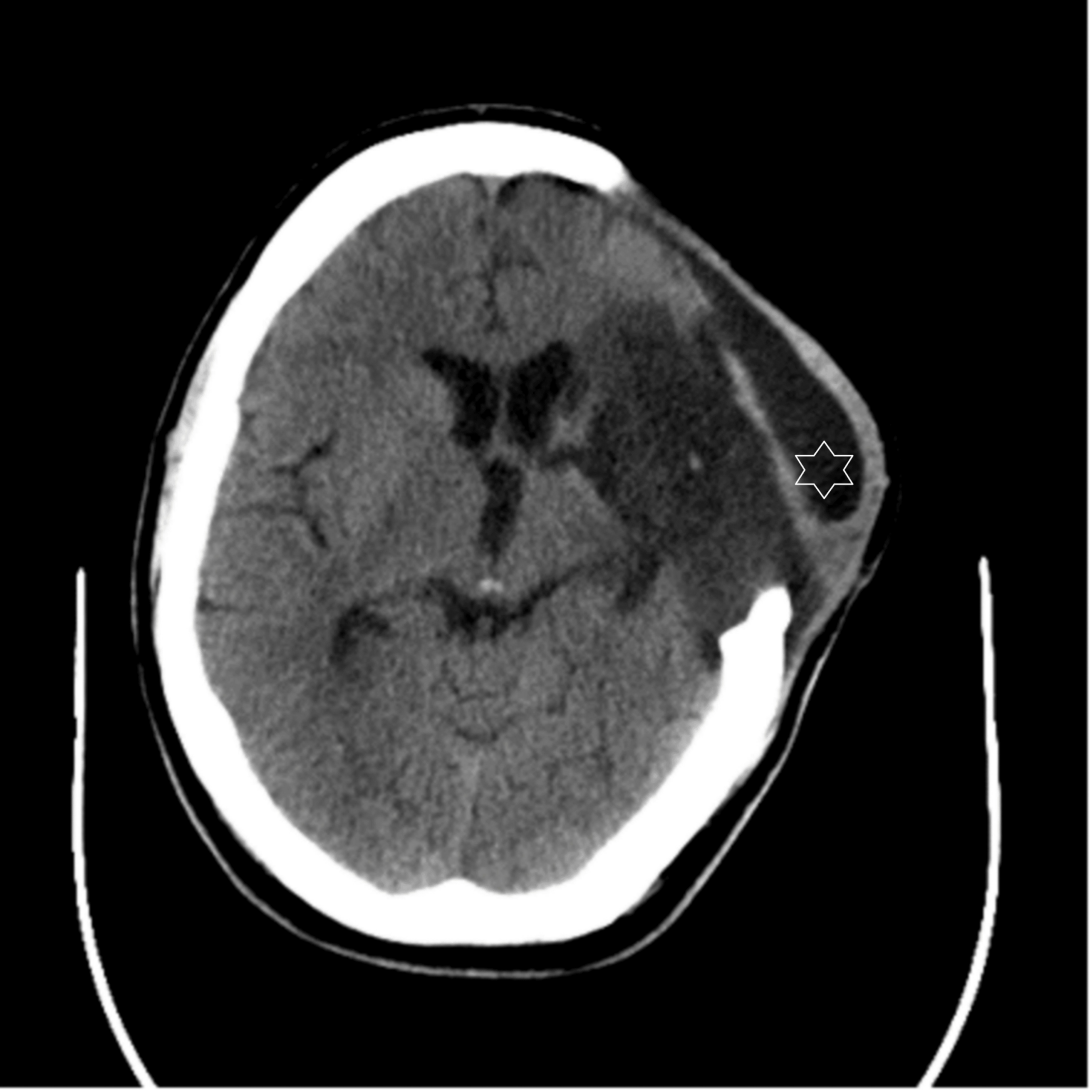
CT brain plain-axial images post lumbar puncture demonstrating reduction in swelling
